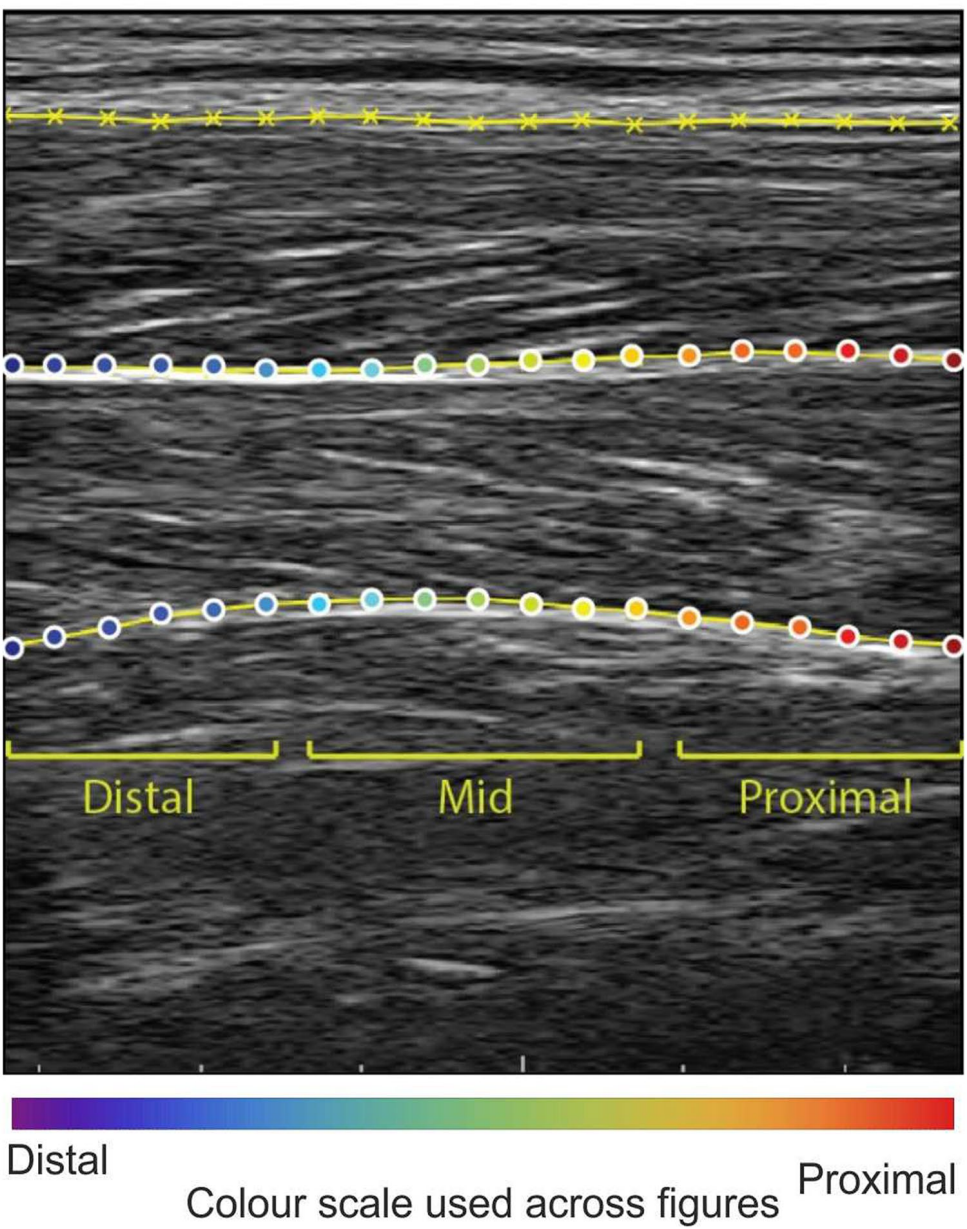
Exemplar ultrasound image with segmentation performed on the superficial and deep aponeuroses of the soleus (SO) muscle during stance phase of steady-state running at 5 m s^−1^. The distal, mid and proximal sections represent the anatomical regions of the points landmarks within the ultrasound image. Example of segmented ultrasound image sequences from this data set can be seen in^16^.
